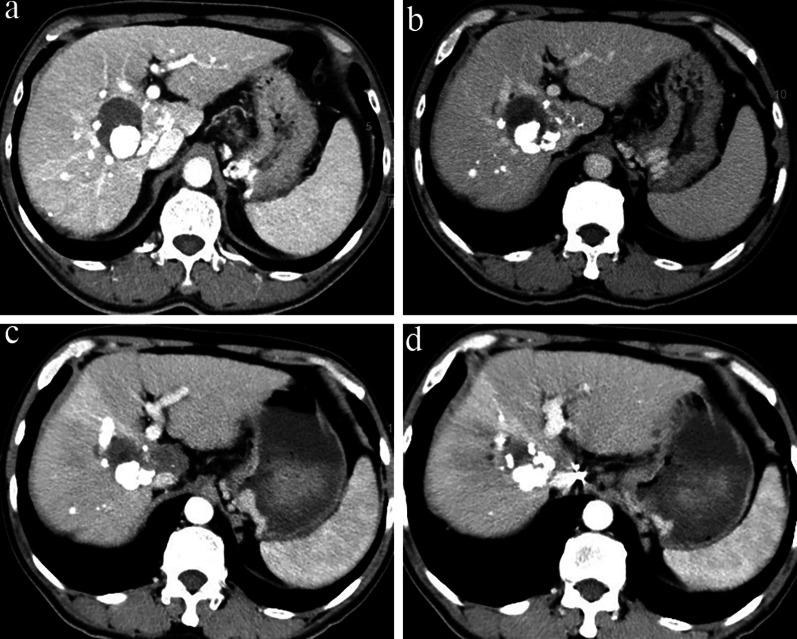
Contrast-enhanced computed tomography (CT) of a 69-year-old man with primary liver cancer (5.6 cm) adjacent to inferior vena cava and portal vein. Contrast-enhanced CT showed abnormal enhancement of liver S1 segment at 1 month after TACE (a). CT-guided MWA was performed but contrast-enhanced CT still showed residual tumor lesion (b) after 1 month. Three months after the CT-guided.^125^I seed implantation, the residual tumor lesions showed no enhancement (c, d)
